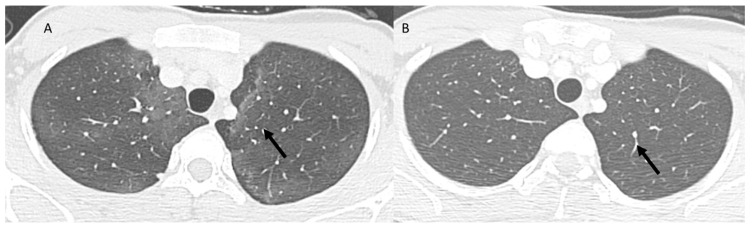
CT assessment (A) of CIP: OP pattern with peribronchovasal and subpleural distribution bilaterally of ground-glass opacities (arrow). Resolution of pneumonitis (B) after steroids (arrow) treatment.
